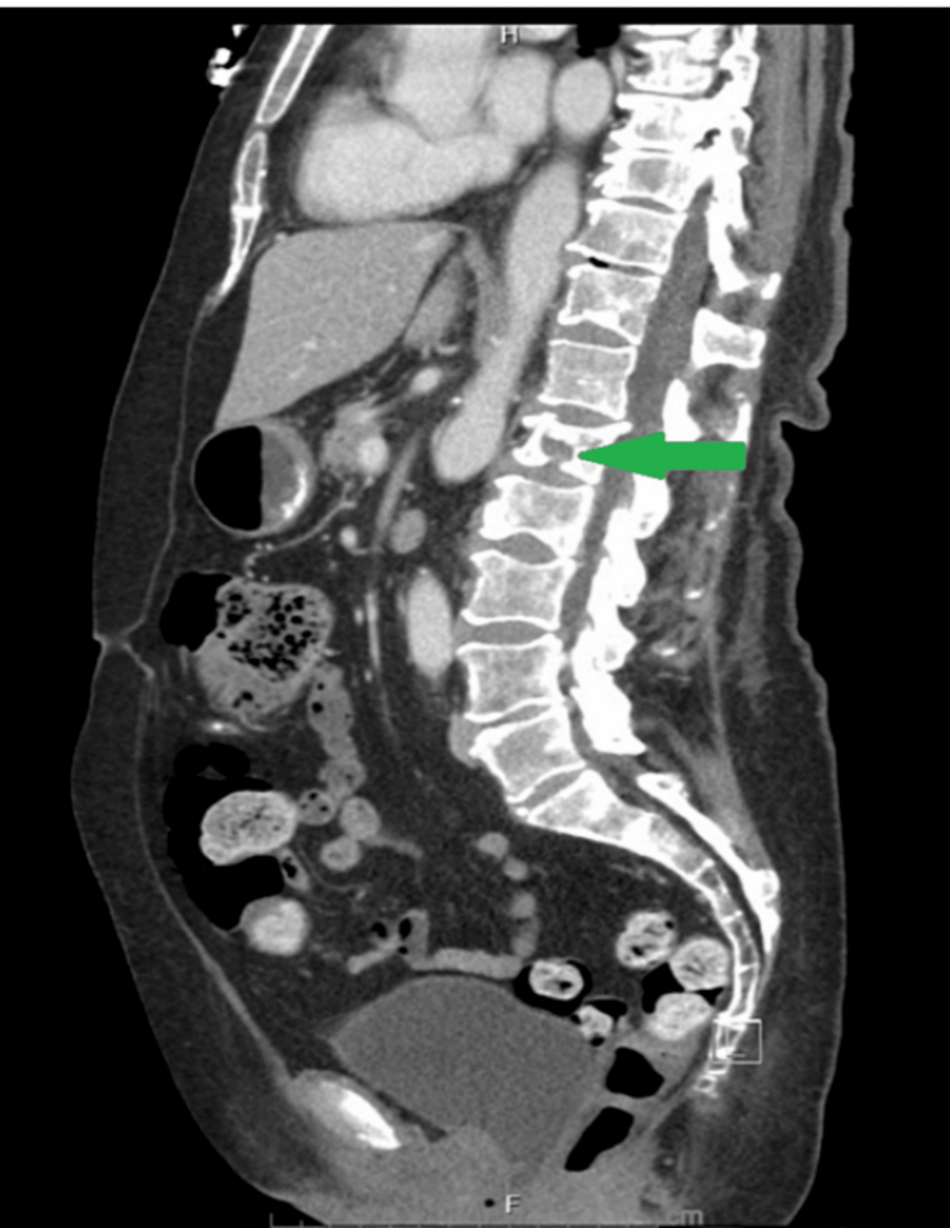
Sagittal CT section of the abdomen and showing L2 compression fracture due to multiple myeloma (green arrow)
